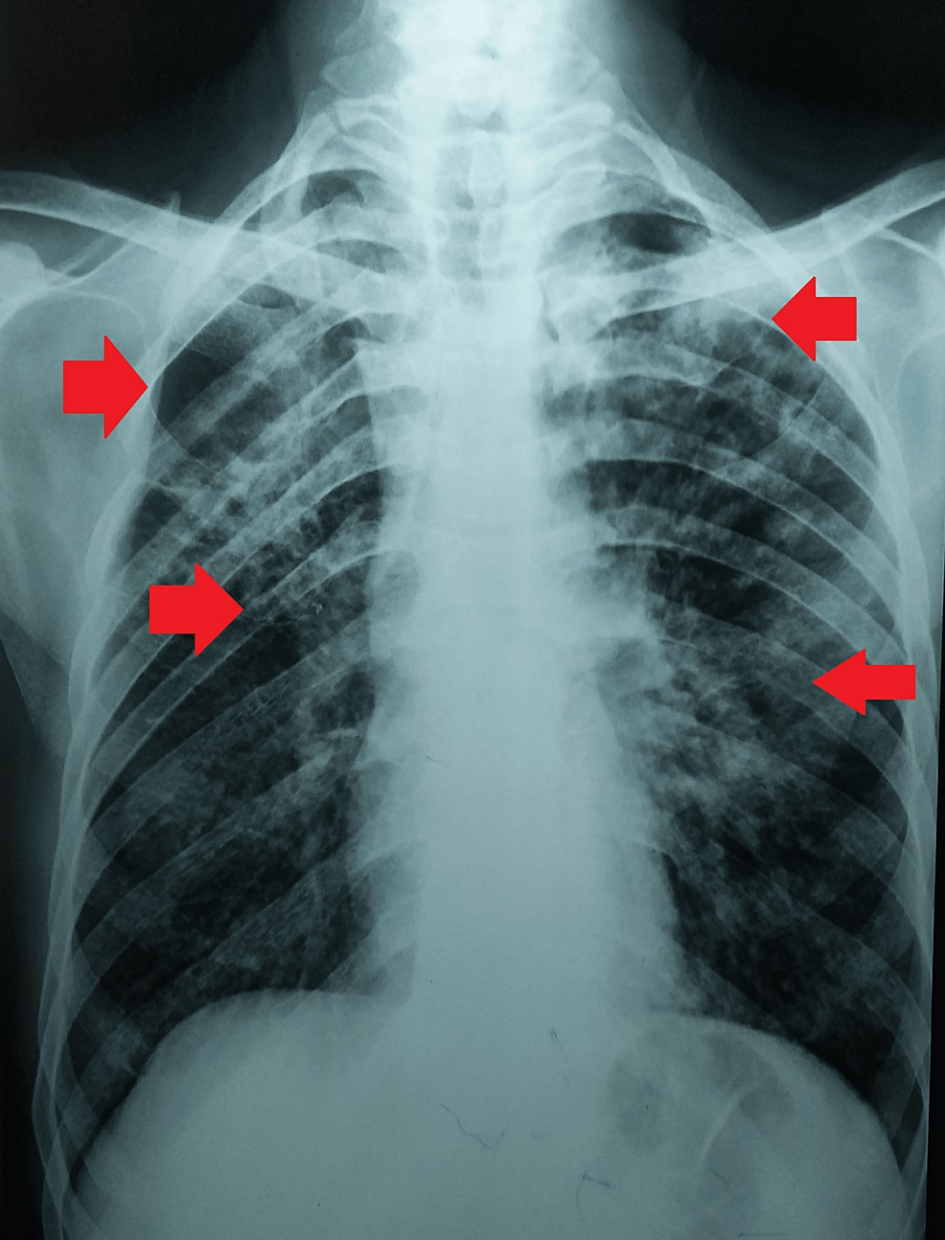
Chest radiograph (PA view) was suggestive of bilateral opacities with a cavity on the right upper lobe PA: posteroanterior
